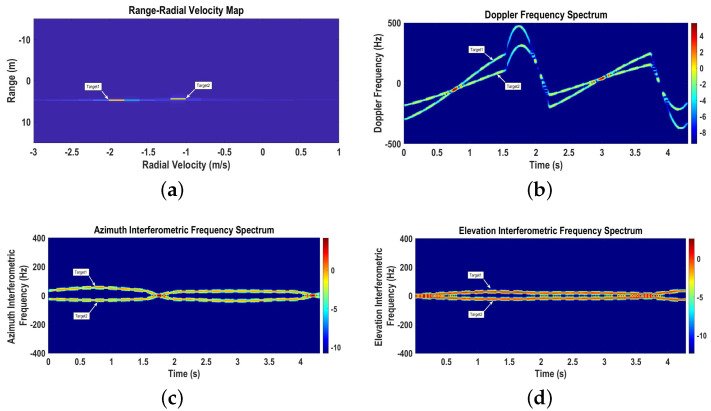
Range–radial velocity map, time-varying Doppler, and azimuth interferometric and elevation interferometric spectrograms for Scenario 2. (a) Range–radial velocity map; (b) Doppler spectrogram; (c) Azimuth interferometric spectrogram; (d) Elevation interferometric spectrogram.
